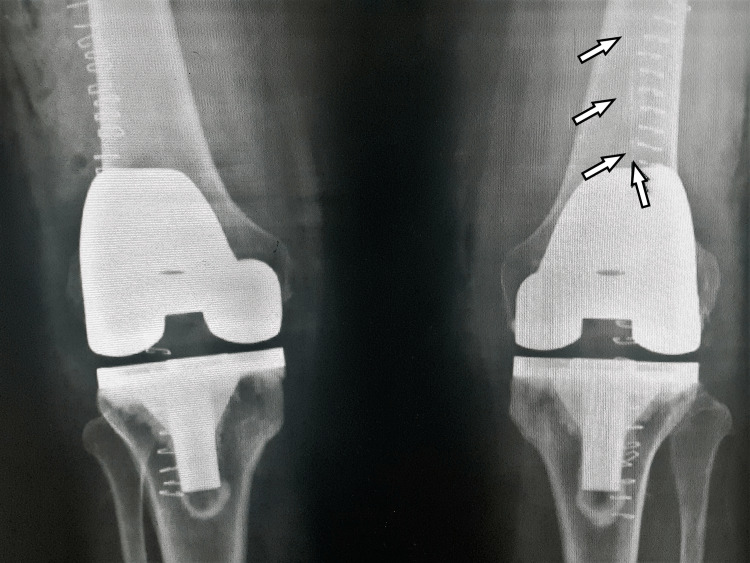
Post-operative radiograph of bilateral knees (anteroposterior view) Post-operative radiograph of bilateral knees in anteroposterior view with a broken drain in left knee marked with white arrows.
